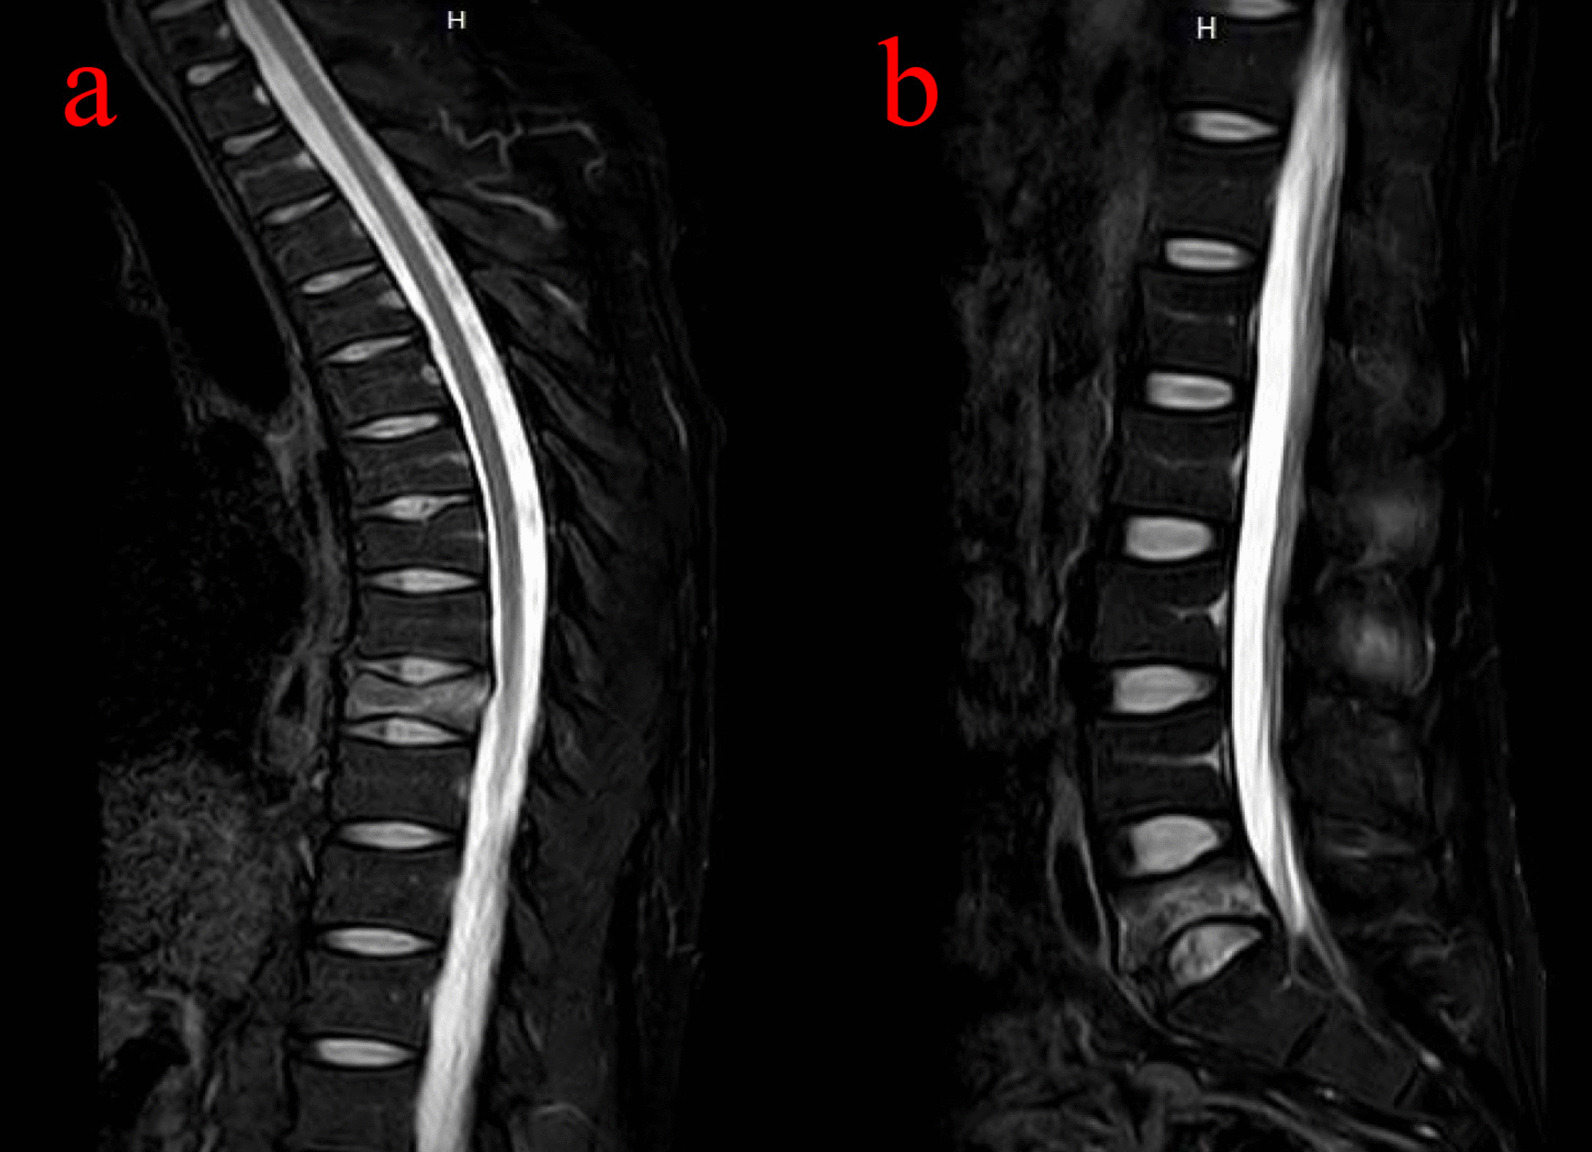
Radiology date of the patient at the time of injury. a Magnetic resonance imaging (MRI) showed T9 VCFs. b MRI showed L5 VCFs
